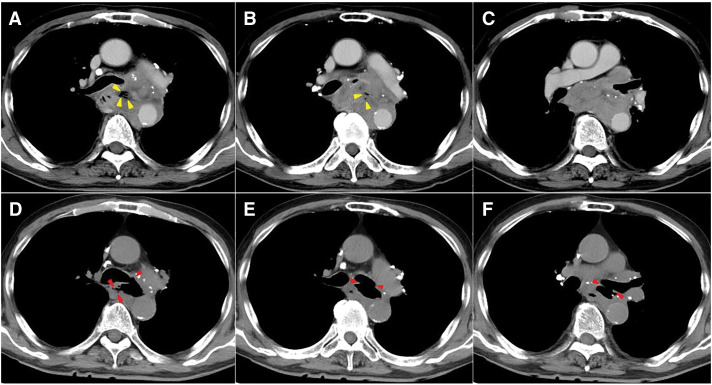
(A–C) CT showed a 10 cm-sized, irregularly shaped space-occupying lesion (SOL) involving the left main bronchus and esophagus. The left main bronchus was obstructed by the SOL, and gas was present within the lesion (yellow arrowheads). (D–F) The tumor shrank remarkably after radiation treatment. However, the esophagobronchial fistula became apparent (red arrowheads).
